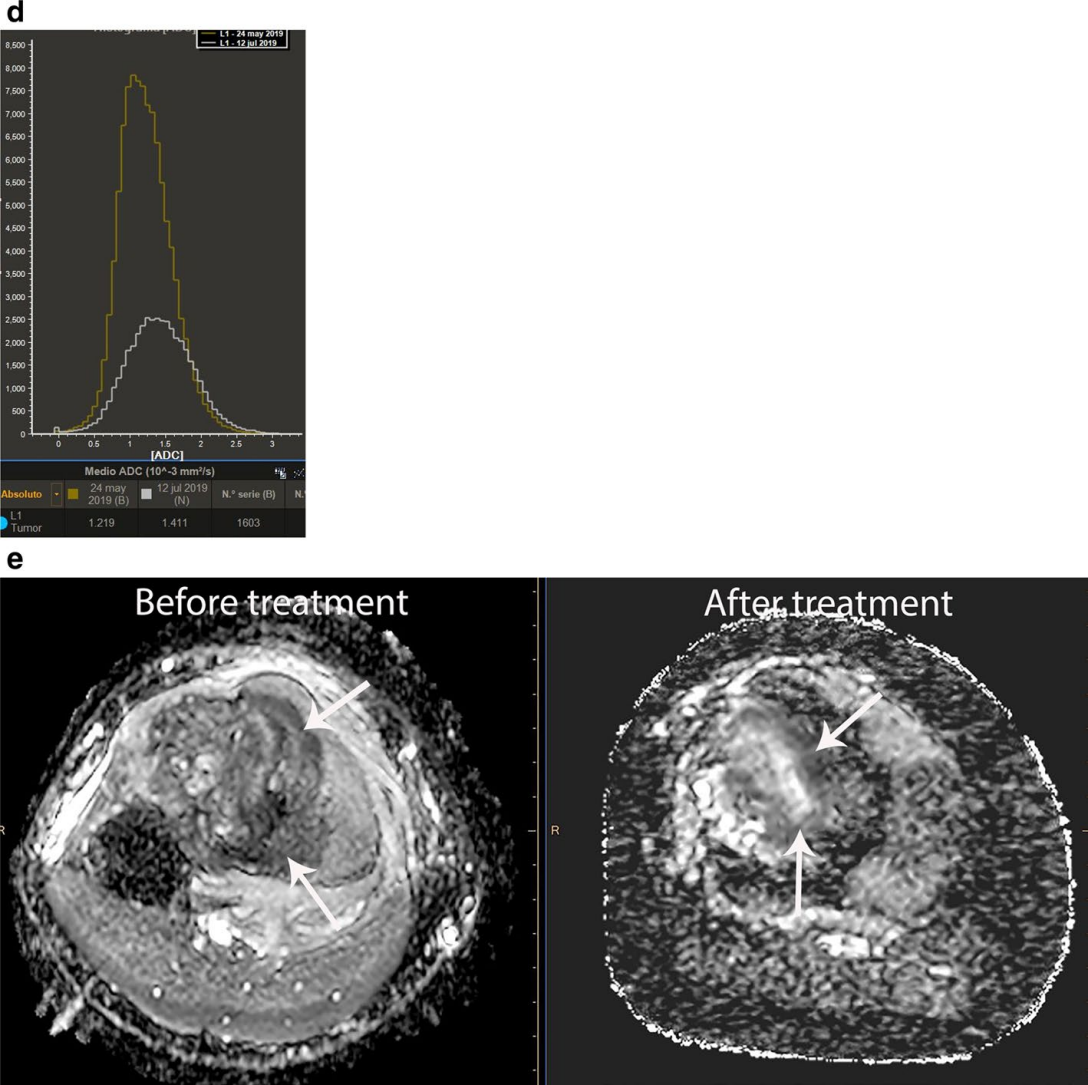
Same patient as in Fig. 3a Axial DCE-MR image (left) with corresponding time intensity curve (TIC) (right) before treatment shows early peripheral enhancement of the tumor (long arrows), which correlates with types III and IV TIC (green curve) and no enhancement of the central part of the tumor (thick arrow), which correlates with a type I TIC (horizontal orange curve), suggestive of necrosis. Peritumoral edema (short arrow) correlates with a type II TIC (pink curve). The blue curve represents the arterial TIC. b Axial DCE-MR image (left) with corresponding TIC (right) after treatment shows enhancement of the residual tumor (short arrow), which correlates with a type III TIC (orange curve). There is no change of the tumor necrosis (green curve) or peritumoral edema (pink curve). c Volumetry and d histogram of ADC maps performed before and after treatment shows an increase of the ADC mean values from 1.22 mm^2/^s to 1.41 mm^2/^s. e) ADC image after treatment shows residual tumor (short arrows)
